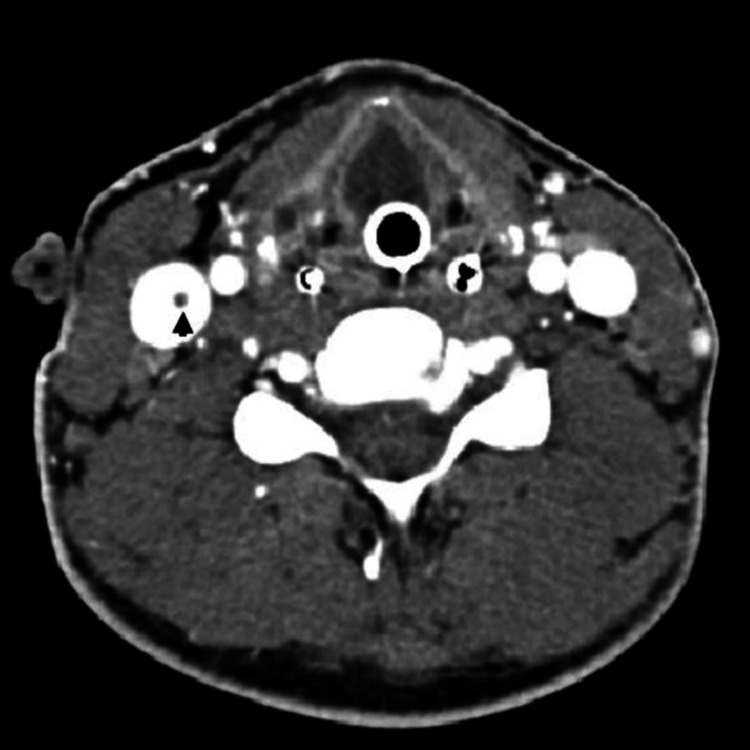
CT neck with IV contrast Axial contrast-enhanced CT of the neck shows a hypodense subocclusive thrombus within the right internal jugular vein (black arrow) CT: computed tomography; IV: intravenous
